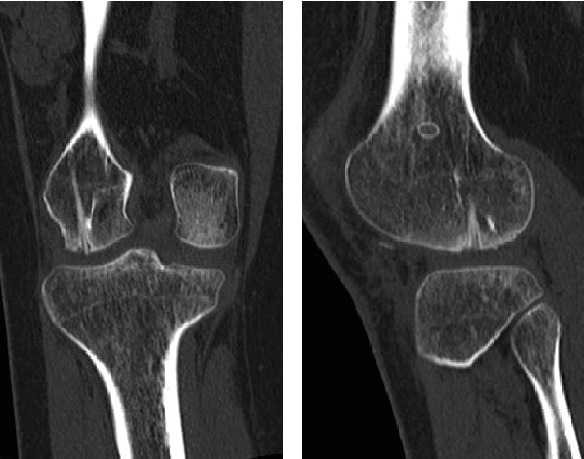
CT images showing good bony fusion and no joint step. (a) Coronal section and (b) sagittal section.
